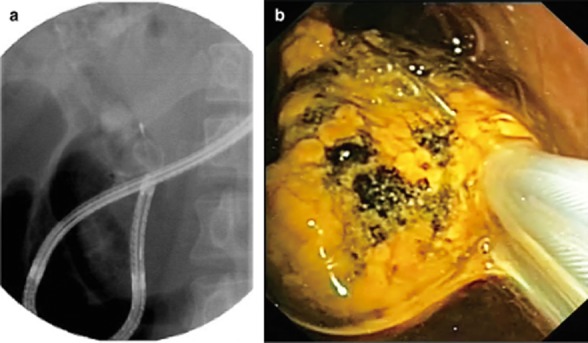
Endoscopic direct lithotripsy. (a) X-ray shows grasping a stone using a basket catheter with the prototype cholangioscope. (b) Endoscopic image shows grasping of the stone with a basket.
